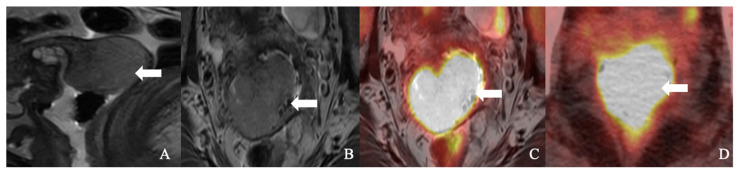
A 51-year-old woman with squamous cell carcinoma of the cervix. (A). Sagittal T2-weighted imaging (T2WI), (B). axial T2WI, and (C). axial fused T2WI positron emission tomography/MRI showing a large (18)F-fluorodeoxyglucose (FDG) avid cervical mass (arrow). (D). An axial positron emission tomography/computed tomography image showed FDG avidity cervical tumor (arrow).
